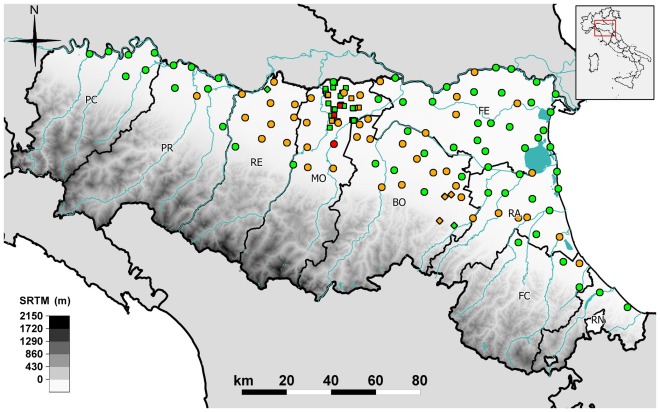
Emilia-Romagna regional map of mosquito sampling stations and locations of PCR–positive pools. Circle, plan trap; square, Modena trap; diamond, extra-plan trap; red, WNV– and USUV–positive station; orange, USUV–positive station; green, negative station. Province abbreviations: PC, Piacenza; PR, Parma; RE, Reggio Emilia; MO, Modena; BO, Bologna; FE, Ferrara; RA, Ravenna; FC, Forlì Cesena; RN, Rimini.
